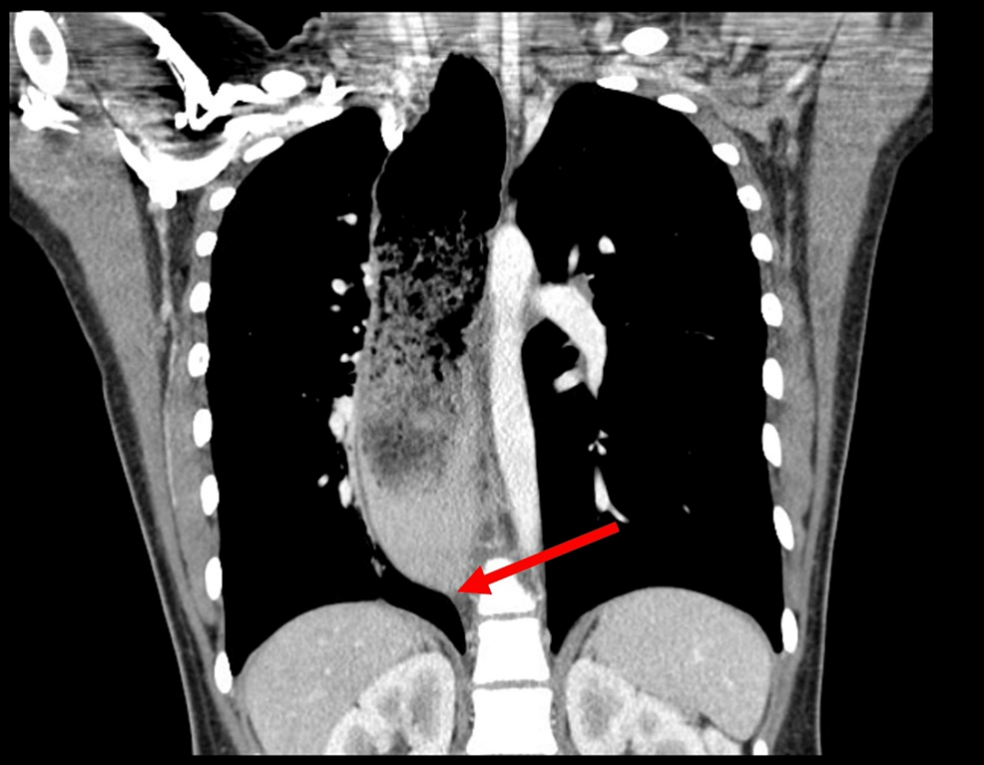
CT Chest Abdomen and Pelvis The red arrow points to the tapering of the esophagus in the gastroesophageal junction region
